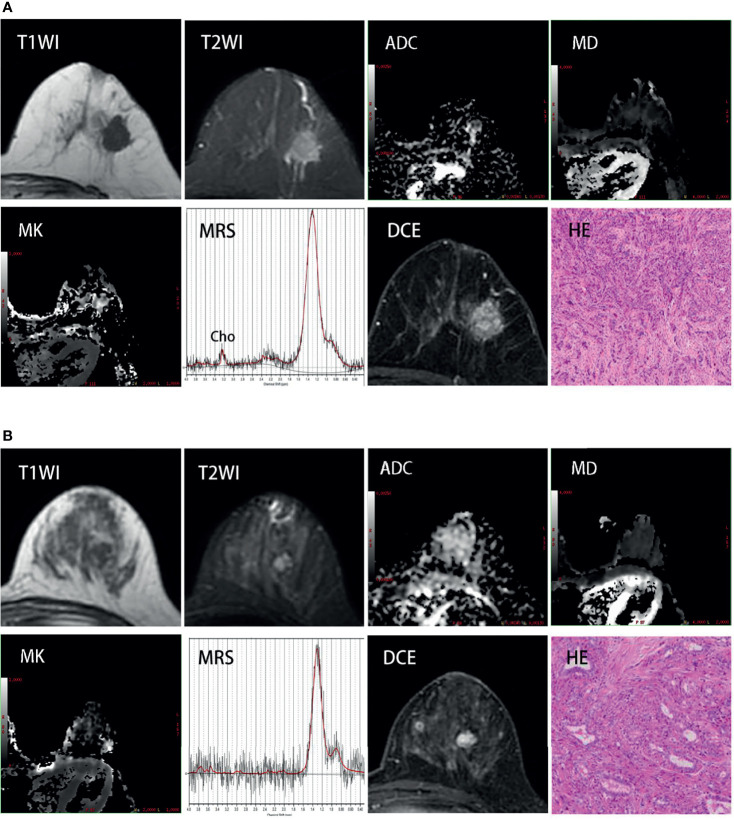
(A) Invasive ductal breast carcinoma grade 3 (estrogen receptor-positive, 98%; progesterone receptor-positive, 98%; HER-2-negative, Ki-67-positive, 90%) in a 58 year-old woman. Images show an unregular lesion of decreased T1 signal and increased T2 signal in the left breast. DCE-MRI shows a mass with unregular borders and imhomogenous enhancement. MK map shows increased signal intensity in this region compared with surrounding glandular (mean:1.867); MD map and ADC map both show decreased signal intensity in the same region (mean:0.919×10^-3^mm^2^/sec and 0.635×10^-3^mm^2^/sec); ^1^H-MRS shows a noticeable Cho peak at 3.23 ppm. (B) Fibroadenoma in a 35-year-old woman. There is an oval lesion with decreased T1 signal, increased T2 signal, regular borders and homogenous enhancement in the left breast. MK map, MD map and ADC map show non-different signal intensity in this region compared with surrounding glandular(mean: 0.243, 1.93×10^-3^mm^2^/sec and 1.613×10^-3^mm^2^/sec); Cho peak doesn’t appear at 3.23 ppm in ^1^H-MRS.
